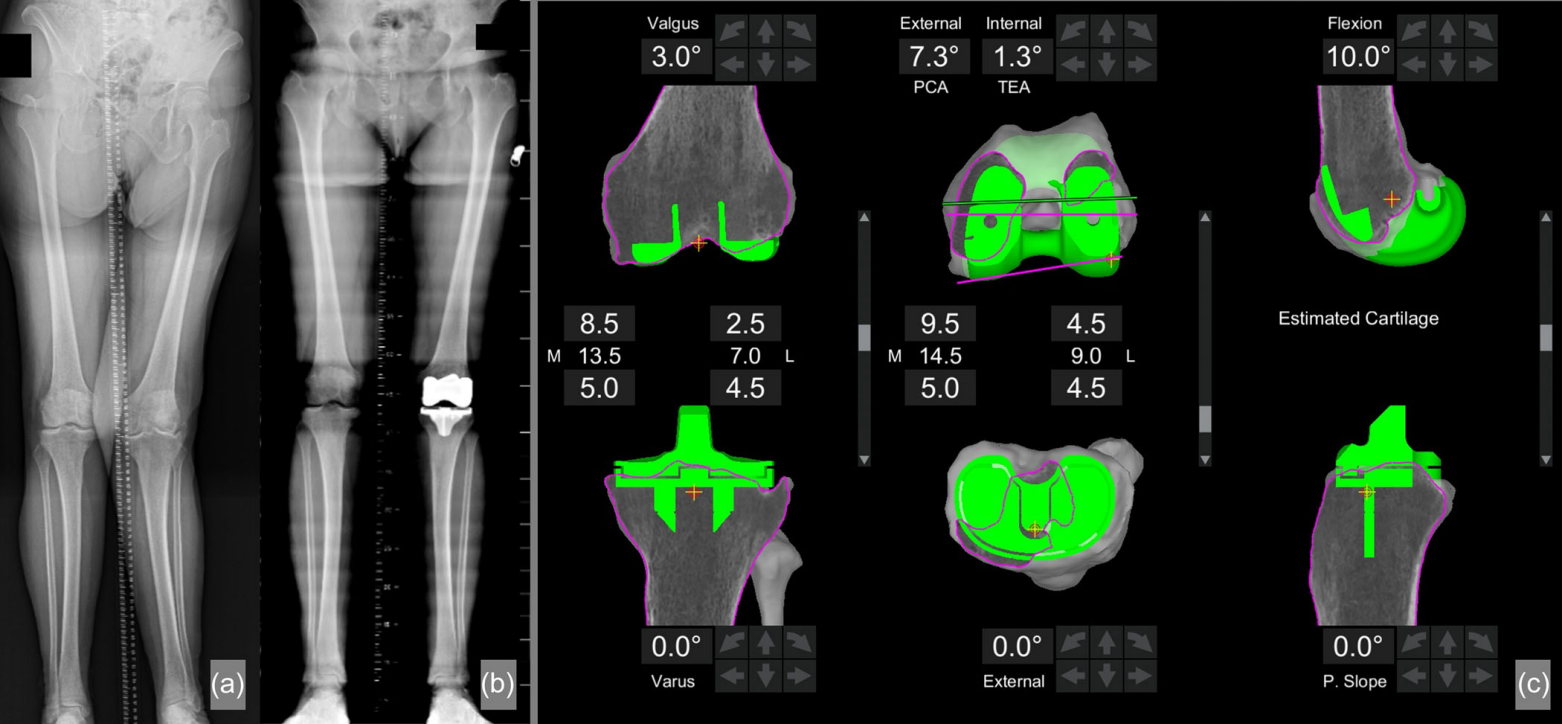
Seventy‐three‐year‐old female patient with a major valgus coronal deformity, presenting a preoperative mechanical hip–knee–ankle (mHKA) angle of 192° on the left side, corrected to 185° after total knee arthroplasty (TKA). (a) Preoperative long‐leg standing radiograph; (b) postoperative long‐leg standing radiograph; (c) intraoperative screenshot of TKA performed with the Mako® robotic system (Stryker®), showing planning adjustments according to functional knee positioning principles.
